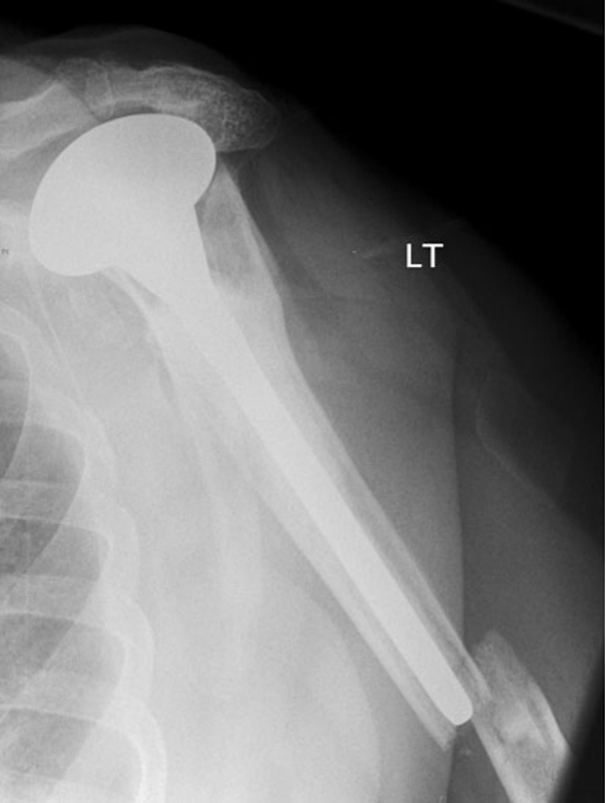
Plain AP radiograph showing Type B2 periprosthetic fracture of left humerus (case 1), with cemented Neer hemiarthroplasty in situ. Note the superior migration of the humeral head and subacromial sclerosis implying pre-existing rotator cuff deficiency as well as the osteoporotic bone of the humerus
